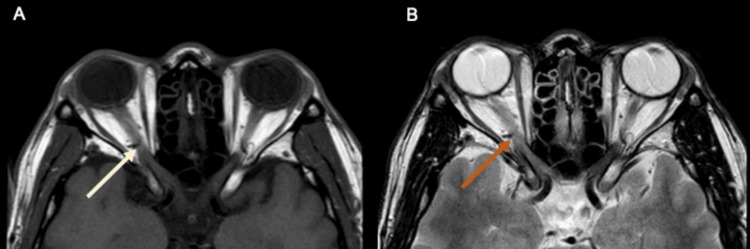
Magnetic resonance imaging of the brain/orbit at T1 (A) and magnetic resonance imaging of the brain/orbit at T2 (B). A: Magnetic resonance imaging of the brain/orbit at T1 showed thickening of the right optic nerve (yellow arrow). B: A thickened right optic nerve with a hyperintensity seen on T2/fluid-attenuated inversion recovery with mild T2 hyperintensity of the myelin sheath (red arrow).
